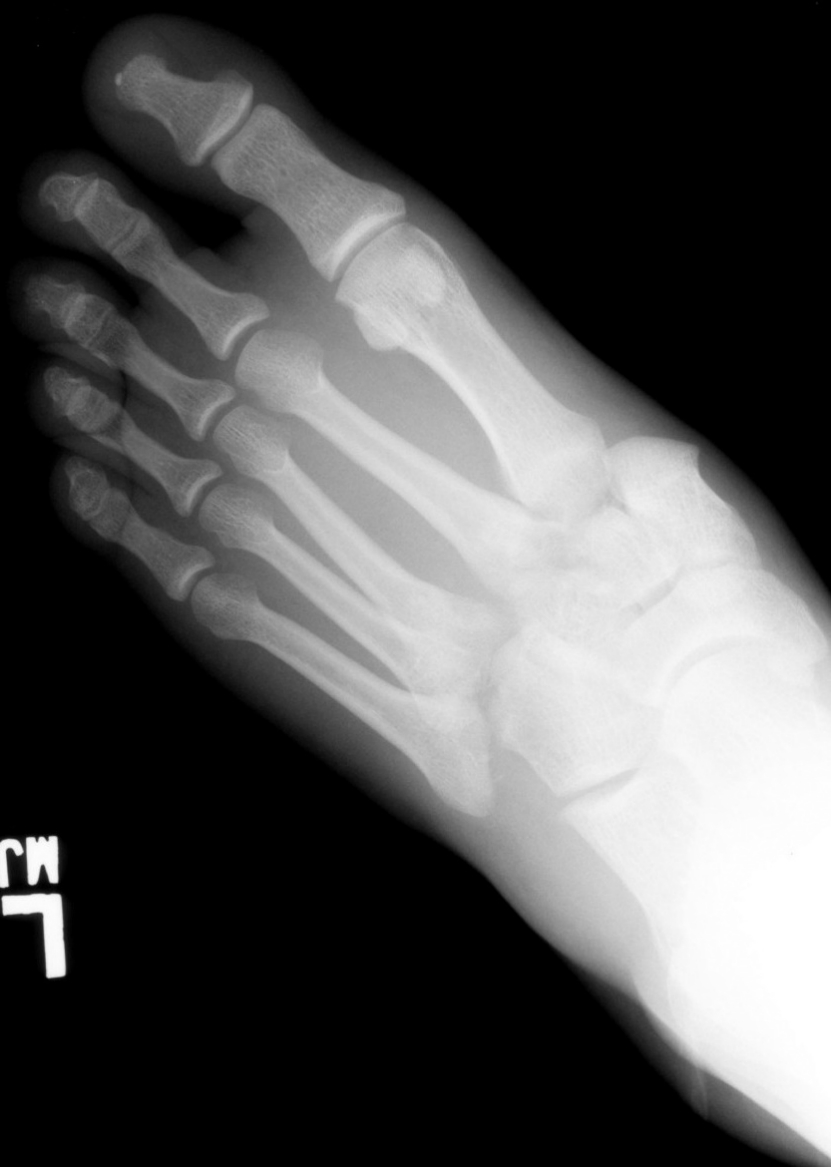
Radiograph of the left foot. There is lateral displacement of the first, second, and third metatarsals (tarsometatarsal or Lisfranc joint) with associated fracture of the middle cuneiform.
